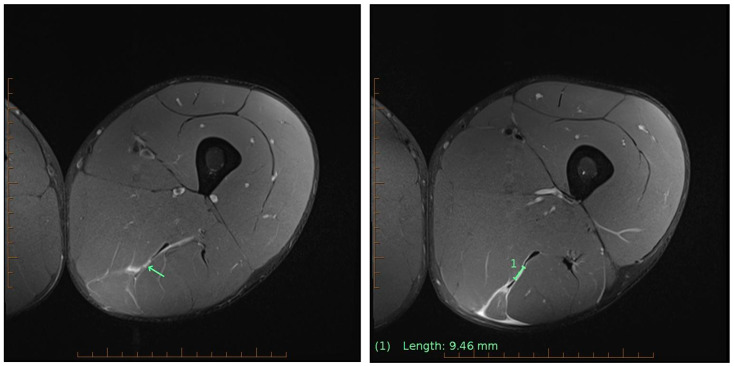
Post-injury magnetic resonance imaging classified the affliction as a BAMIC Grade 2b hamstring injury. Green arrows indicate key prognostic features.
